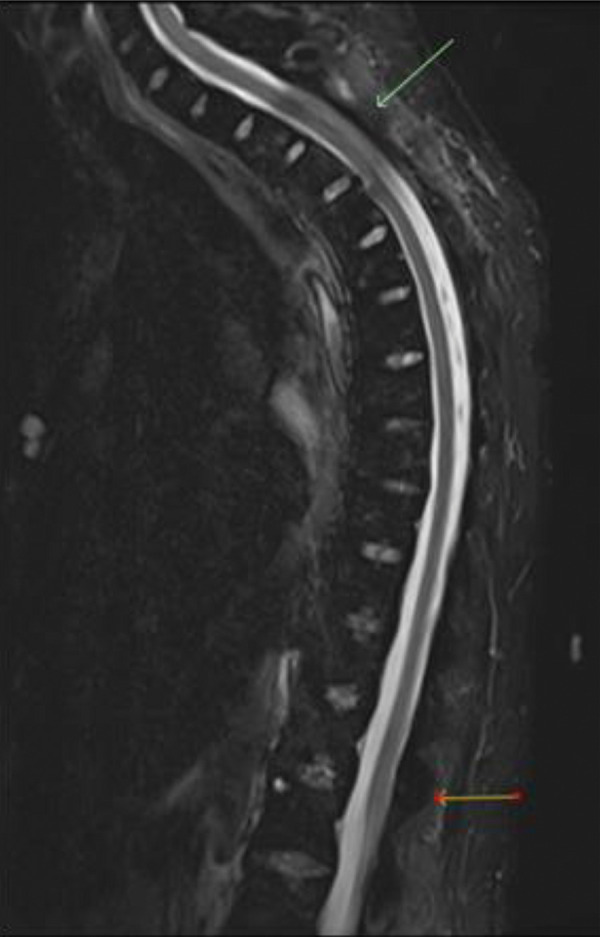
Repeat MRI 3 months after resection of the T3 lesion and PTX shows stable resection site at T3 and regression of T12 tumor.
